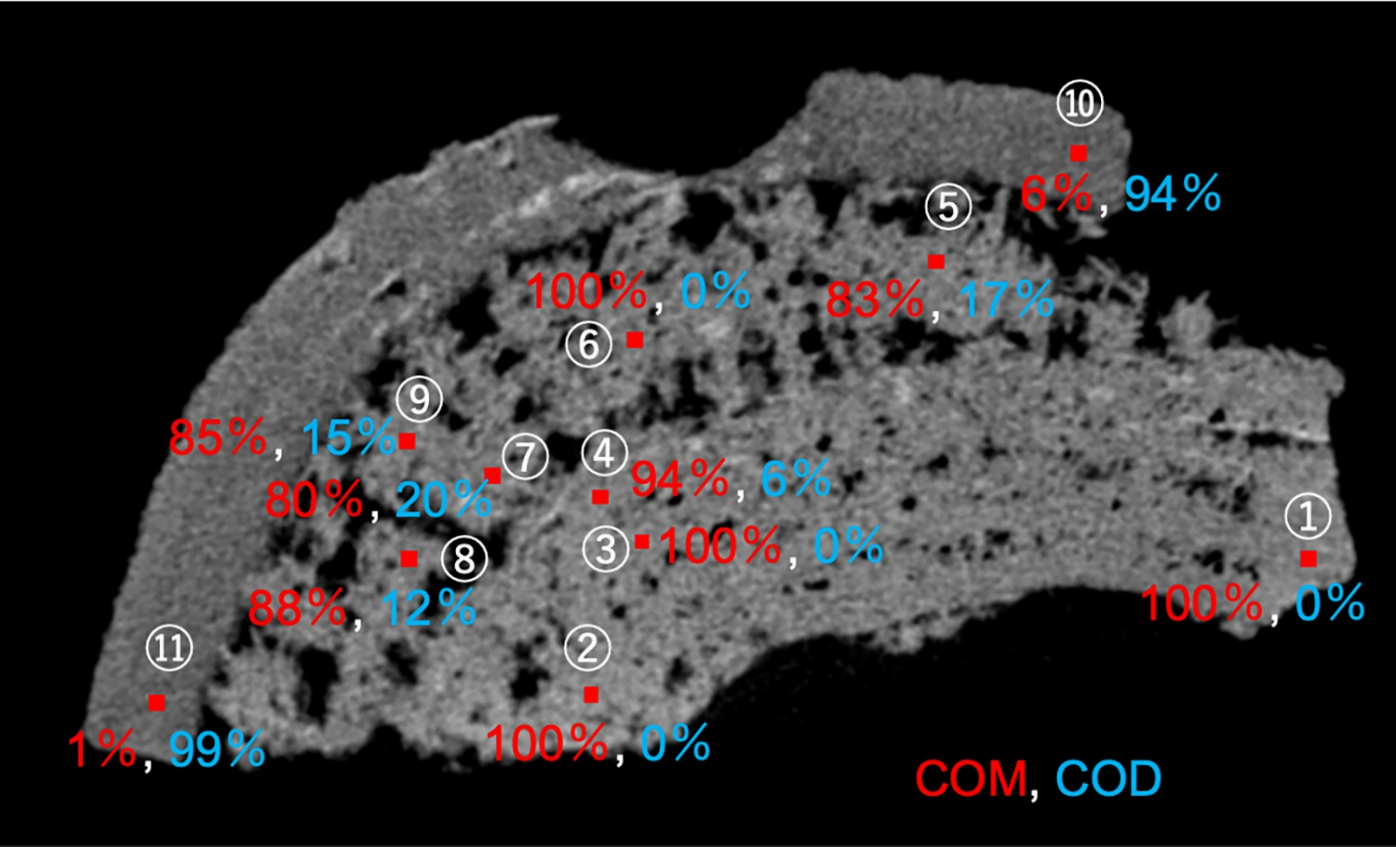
A microfocus X-ray CT image. Eleven analysis points are indicated with *red solid squares* and *numbers*. The analyzed results (the ratio of COM and COD in the 50-mm^2^ squares) are also shown. *Red numbers*: the COM ratio. *Blue numbers*: the COD ratio for each area.
